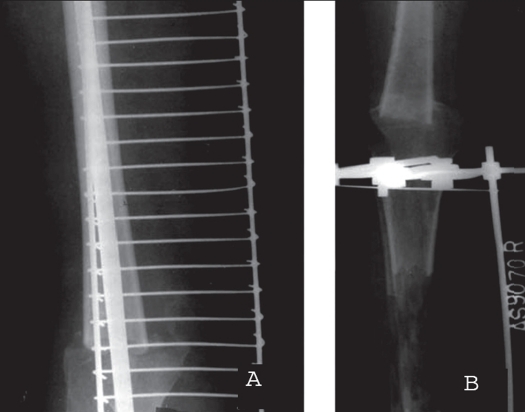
A) X-ray showing segmental resection of bone and telescoping of the fragments over an intramedullary nail. B) Tibial lengthening being performed after resection arthrodesis was achieved.
